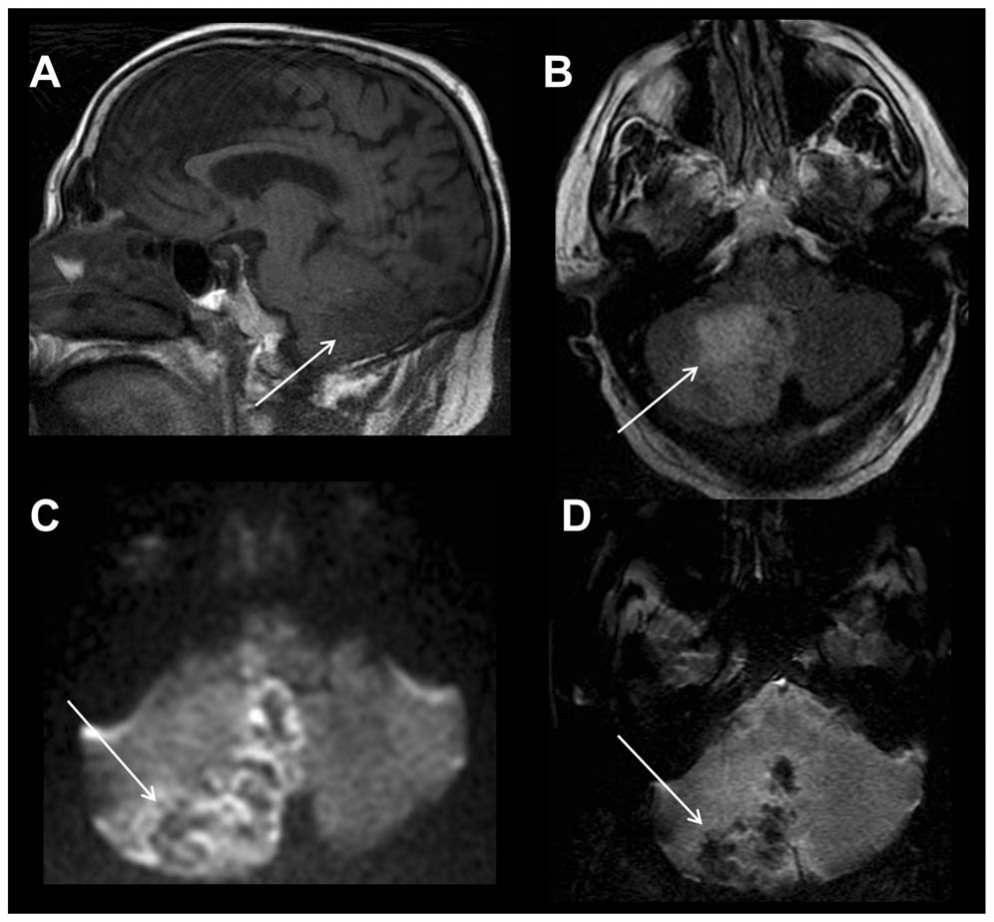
Hemorrhagic acute cerebellar infarction in a 79 year old male with a focal neurological deficit. Sagittal T1-weighted image (A) shows hypointense signal within the right inferior cerebellar hemisphere (white arrow). Axial T2-weighted FLAIR image (B) demonstrates geographic hyperintense signal and mass effect within the right inferior cerebellar hemisphere (white arrow). Axial diffusion weighted trace image (C) shows restricted diffusion in the right cerebellar hemisphere compatible with infarction (white arrow) as well as areas of hypointensity reflecting hemorrhage. Axial gradient recalled echo image shows susceptibility effect within the right cerebellar hemisphere, which is consistent with hemorrhage (white arrow).
